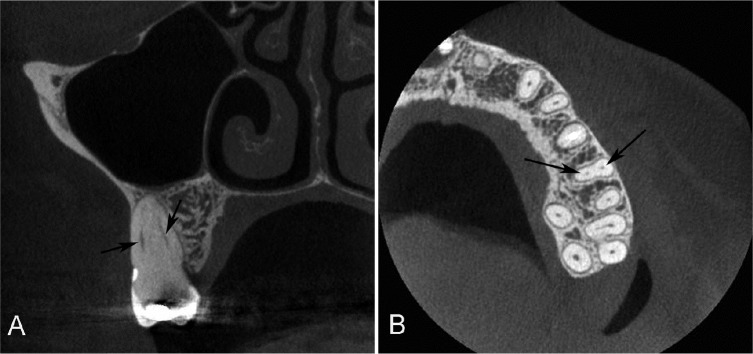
Cone-beam computerized tomography (CBCT) images of maxillary first molars with 2 canals (black arrows) in the mesiobuccal root as viewed in the coronal and axial directions. *A)* Coronal view with the MB root exhibiting type II canal configuration; *B)* Axial view
